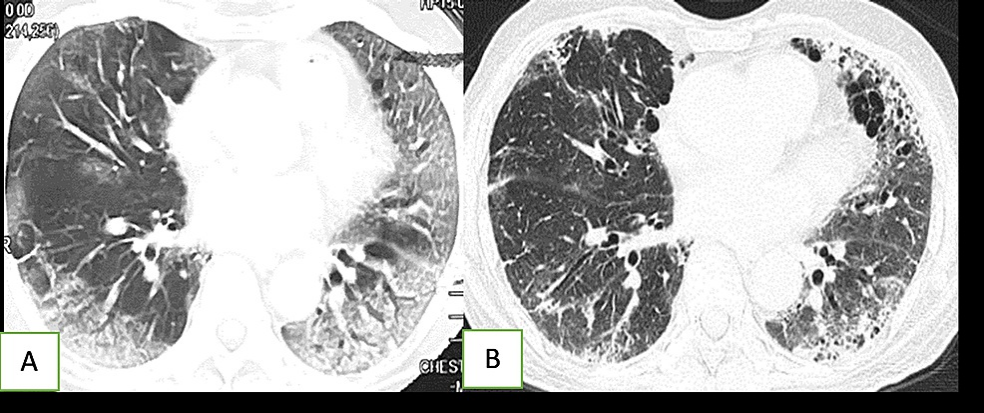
A 76-year-old man with severe COVID-19 Initial CT at presentation shows bilateral ground-glass opacities (GGOs) with peripheral predominance (A). Follow-up CT after 2.5 months shows resolution of the GGOs, and the involved areas are now showing cystic changes and some linear opacities; note that both scans have mild prominence of bronchi, which show a ‘wavy’ contour. In the second image, the cysts are not of honeycomb lung that is classical of fibrosis but can be traced to bronchial tree, however, the presence of some GGOs next to the cysts gives it the appearance of typical honeycomb lung as seen in the fibrosing conditions such as usual interstitial pneumonia (B).
